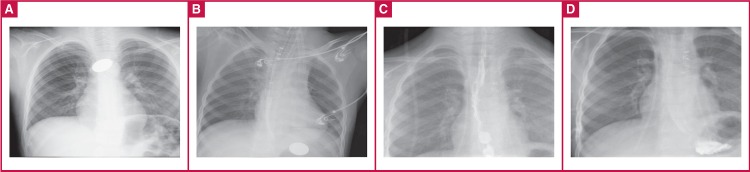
A. Chest radiography showing the first position of the ingested coin. B. Reposition of the coin in the stomach and visualisation of the achalasia balloon. C, D. Oesophageal radiographs on postoperative day 12.
